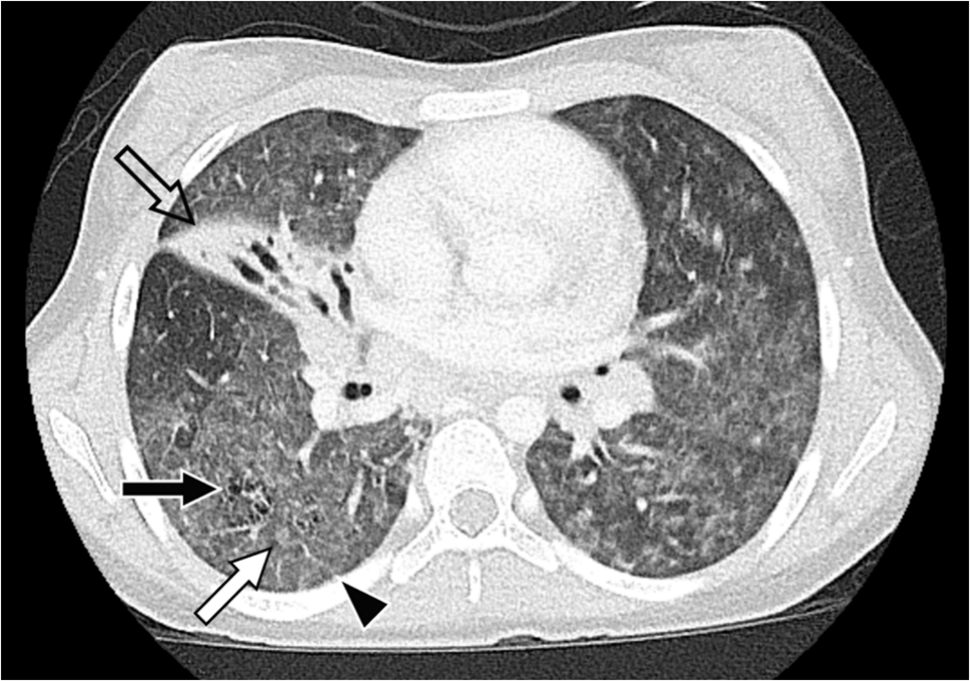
14-year-old female with Sjogren syndrome. Axial CT image with contrast showing extensive ground-glass opacity, peribronchovascular cysts (black arrow), ill-defined centrilobular nodules (white arrow), and interlobular septal thickening (arrowhead) suggesting lymphoid interstitial pneumonia. Right middle lobe collapse and bronchial dilation are also present (open arrow)
